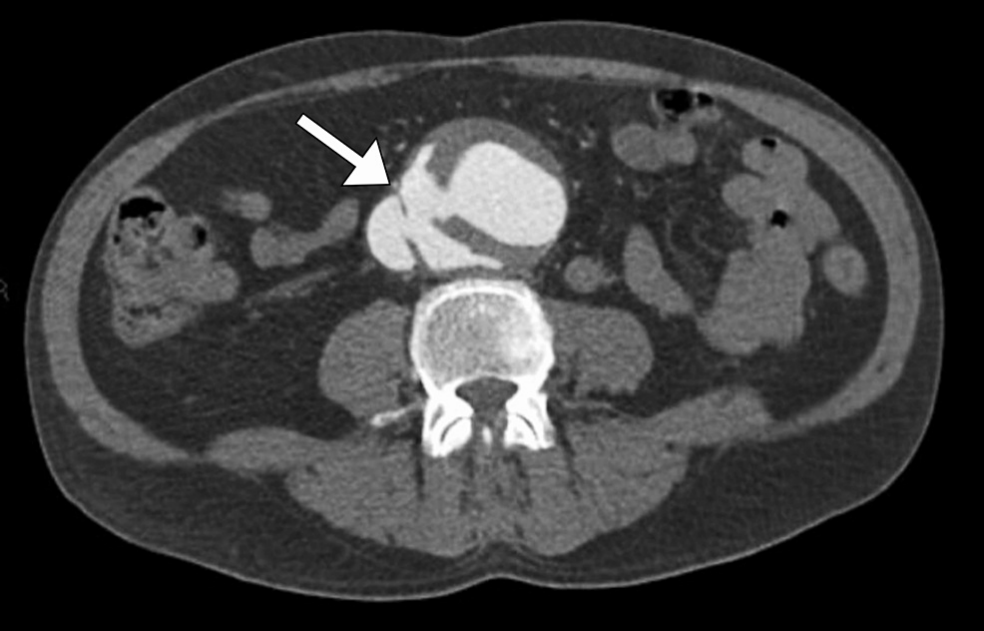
Axial CT image demonstrating an aneurysmal dilatation of the infrarenal abdominal aorta and an abnormal connection between the aneurysm and the inferior vena cava (arrow)
